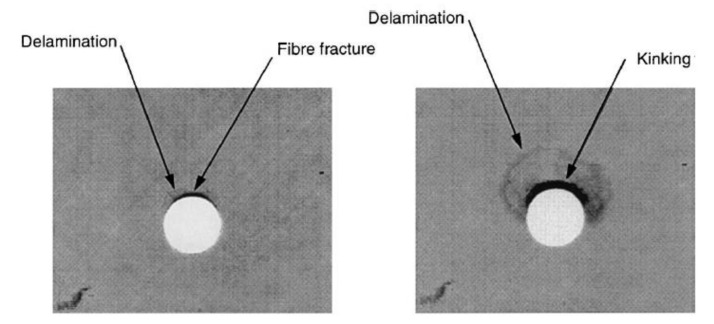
X-ray images of carbon/epoxy bolted joints after loading to different levels and disassembling [133].
